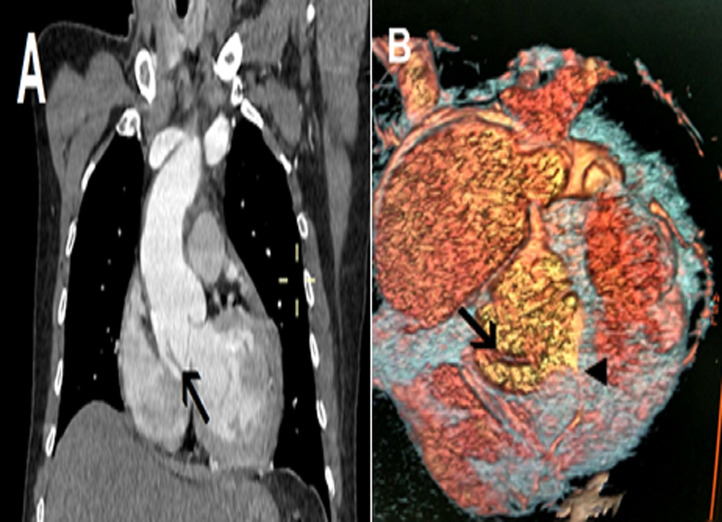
aortic CT angiogram showing Stanford type A aortic dissection: (A) coronal section showing intimal dissection flap (arrow) and pericardial effusion; (B) three dimensionnally reconstructed image of aortic root, showing dissection flap, wich involve the right coronary artery (arrowhead)
